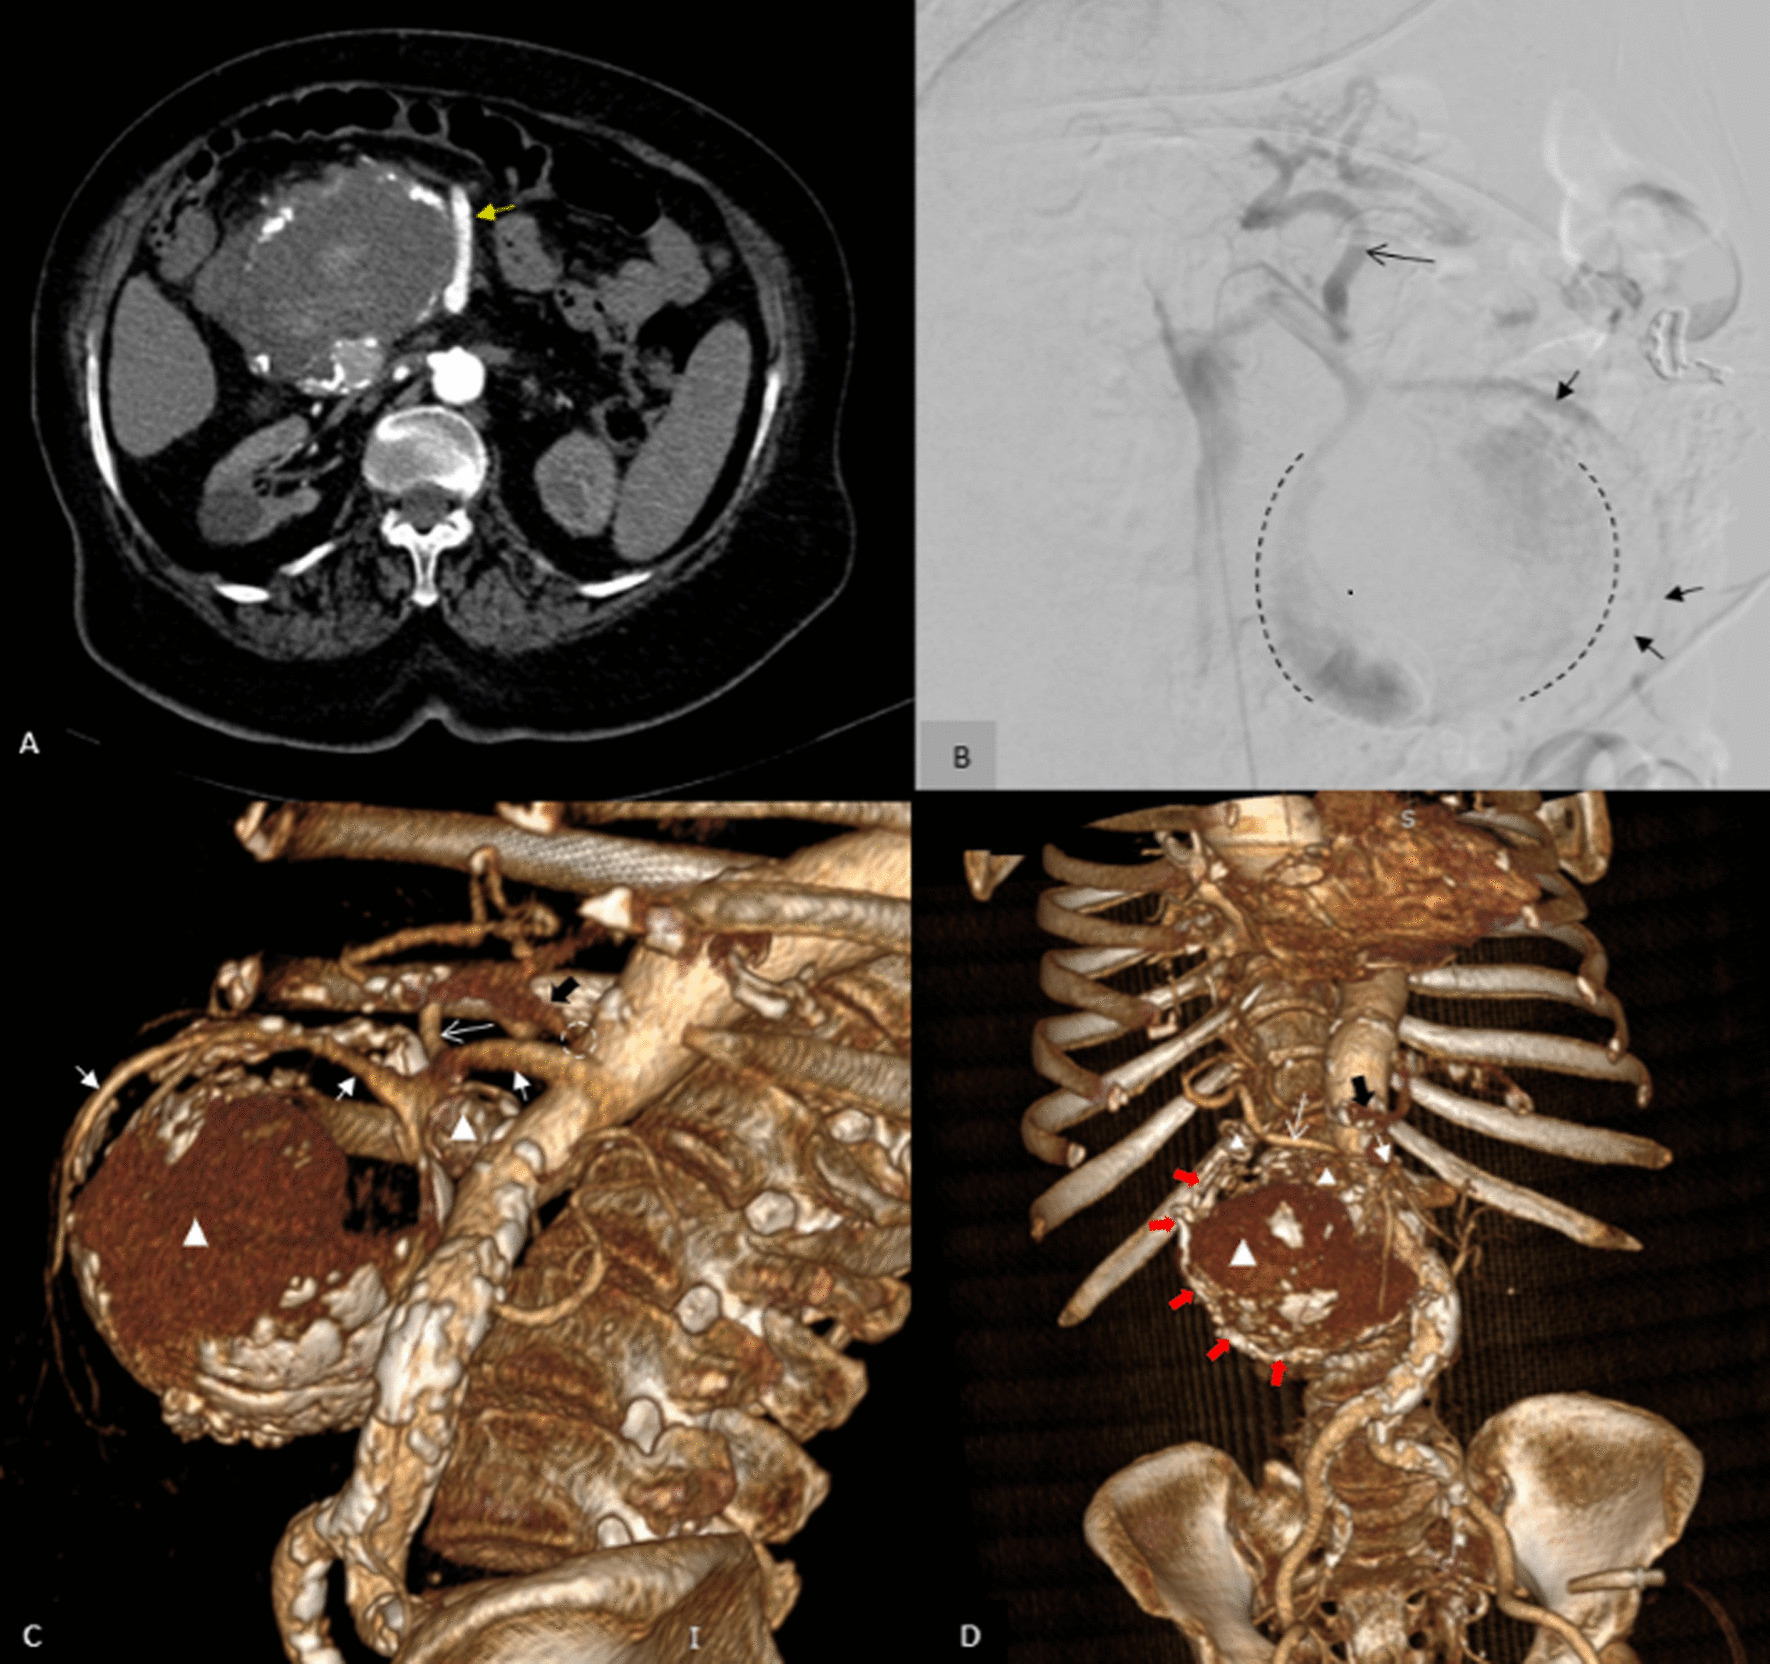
A The yellow arrow in the axial plane of computed tomography angiography indicates superior mesenteric artery, which continues its course to branches to supply small bowel. B Conventional angiography. Huge aneurysm originating from posterior and inferior surface of the proximal of superior mesenteric artery is seen in the lateral view of the superior mesenteric artery angiogram. The margin of this aneurysm is shown by the black dashed line. Short black arrows indicate the superior mesenteric artery itself, which is continuously branched in front of this aneurysm. A long black arrow indicates the right hepatic artery originating from superior mesenteric artery. C, D Reconstructed volume rendering images. White arrowheads point to three aneurysms, and short white arrows indicate superior mesenteric artery and its branches. Long white arrow indicates the right hepatic artery originating from superior mesenteric artery. Solid red arrows indicate anterior pancreaticoduodenal artery’s course which originates superiorly from gasrtoduodenal artery and is connected inferiorly to the huge aneurysm. A solid black arrow indicates the celiac trunk. The dotted circle shows obvous narrowing of the origin of the celiac trunk
